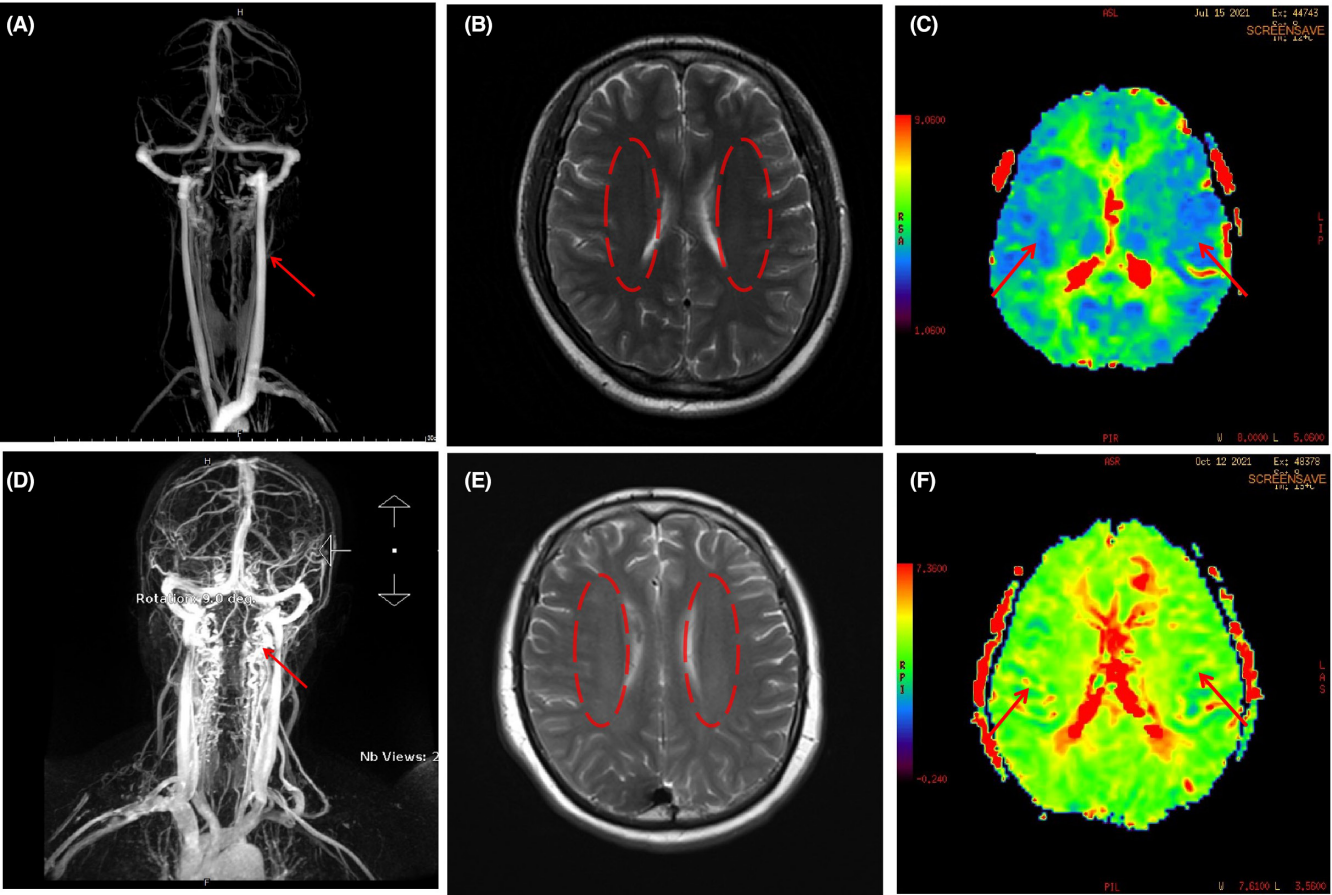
IJV slenderness and stenosis on CE‐MRV/ MRI T2W‐FLAIR /PWI. IJV slenderness (red arrow): A: CE‐MRV without surrounded collateral veins, B: MRI T2W‐FLAIR no cloudy‐like white matter hyper‐intensity (WMH), C: PWI showed normal perfusion, IJV stenosis (red arrow): D: CE‐MRV showed local stenosis surrounded by more collateral veins, E: MRI T2W‐FLAIR showed remarkable cloudy‐like WMH, F: PWI showed bilateral hypo‐perfusion
